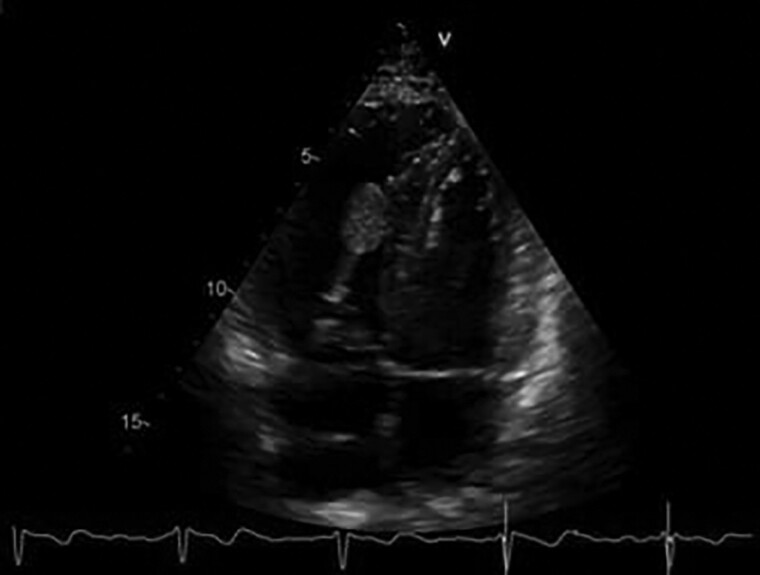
Transthoracic cardiac echocolordoppler showing the reduction of thrombus area on pacing lead in right ventricle (area: from 2.9 to 2.0 cm^2^) after 1 week of anticoagulant therapy.
